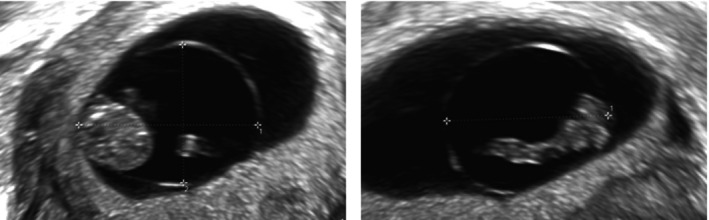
Ultrasound images of the amniotic sac at 8 weeks' gestation, showing three perpendicular measurements which were obtained in the longitudinal and transverse planes.
